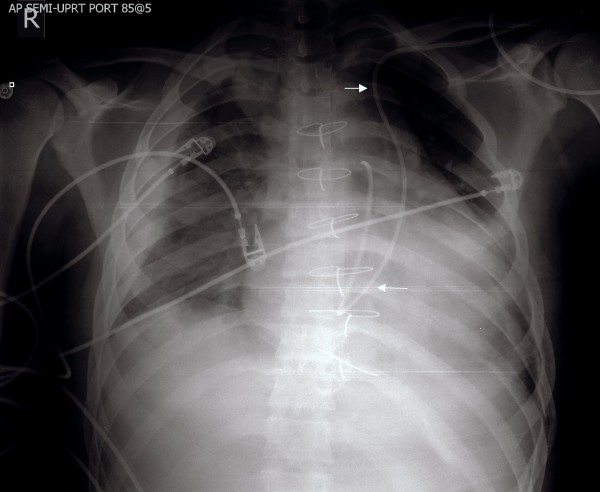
Chest-x-ray demonstrating unusual course of Swan-Ganz catheter (arrows) with its distal end in the proximal pulmonary artery. Note the course of the catheter into the heart on the left side of the spine, rather than the right side via the normal anatomic position of the superior vena cava.
